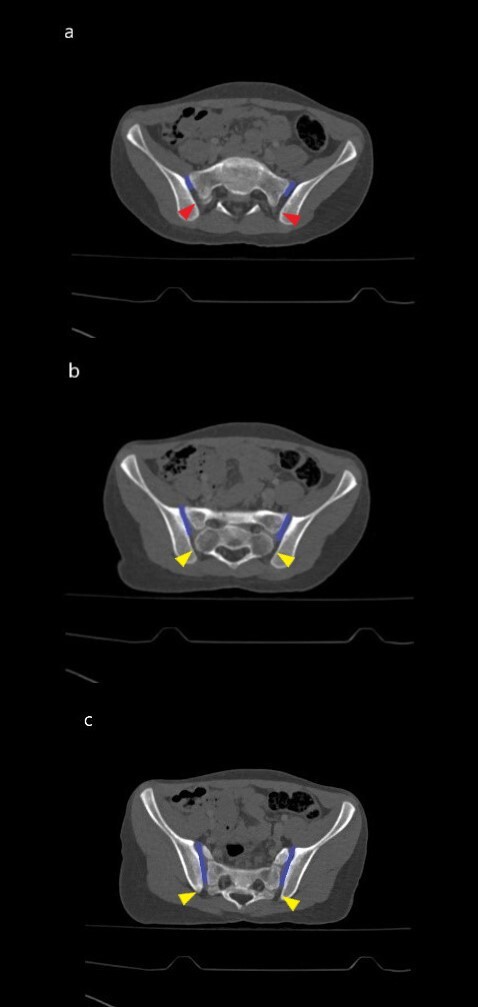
In axial CT images Anterior small part is synovial (blue line); the posterior big part is syndesmosis (red arrowhead) in the taken images of the upper part of joint b. In the midsection of the joint, the anterior half of the joint is synovial (blue line) whereas the posterior half is symphysis-like (yellow arrowhead) c. The anterior big part of the joint is synovial (blue line), only the small posterior part is symphysis-like in the lower part of the joint (yellow arrowhead).
